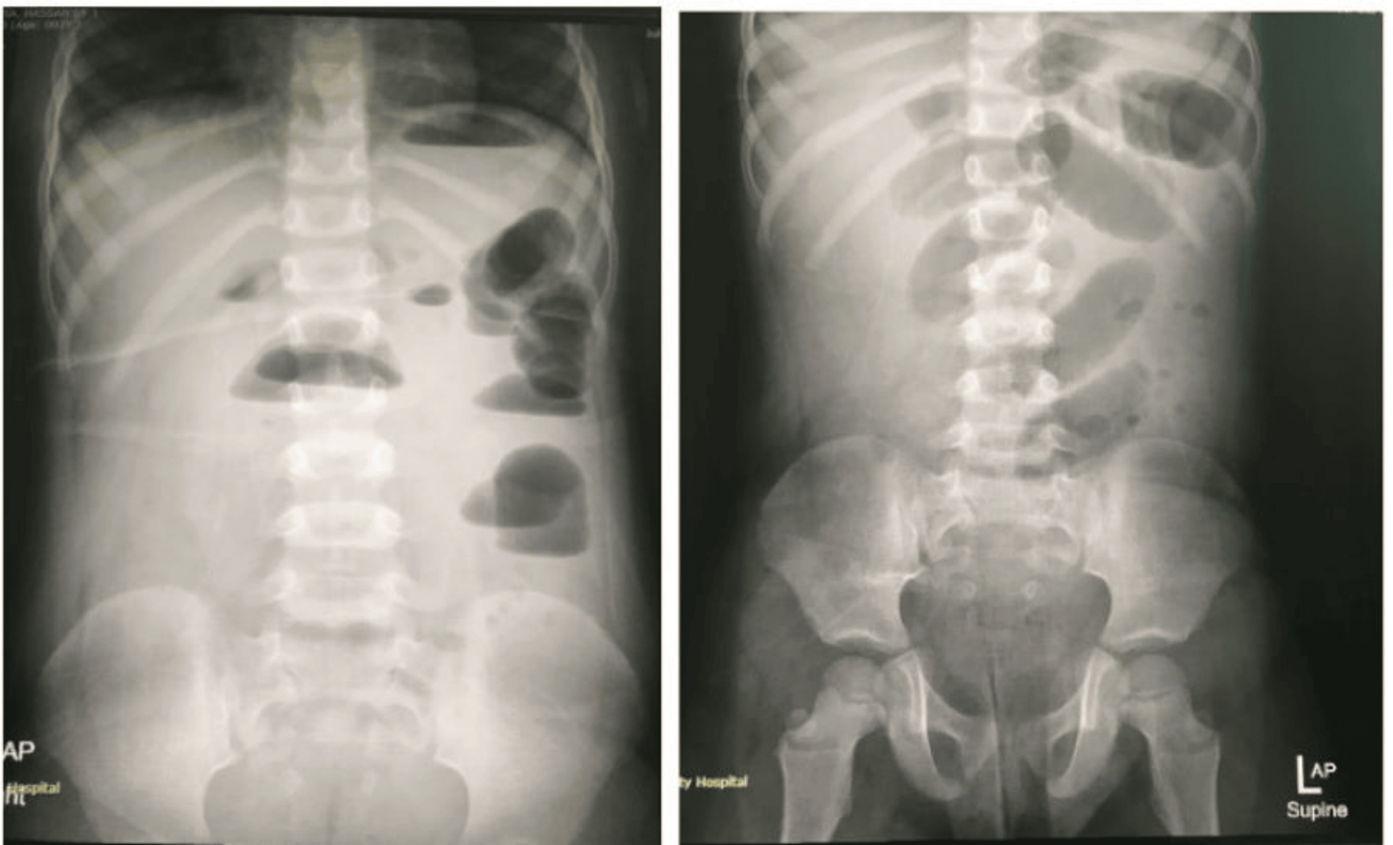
Day 1 abdominal X-rays (erect and supine) show dilated small bowels with multiple air fluid levels.
